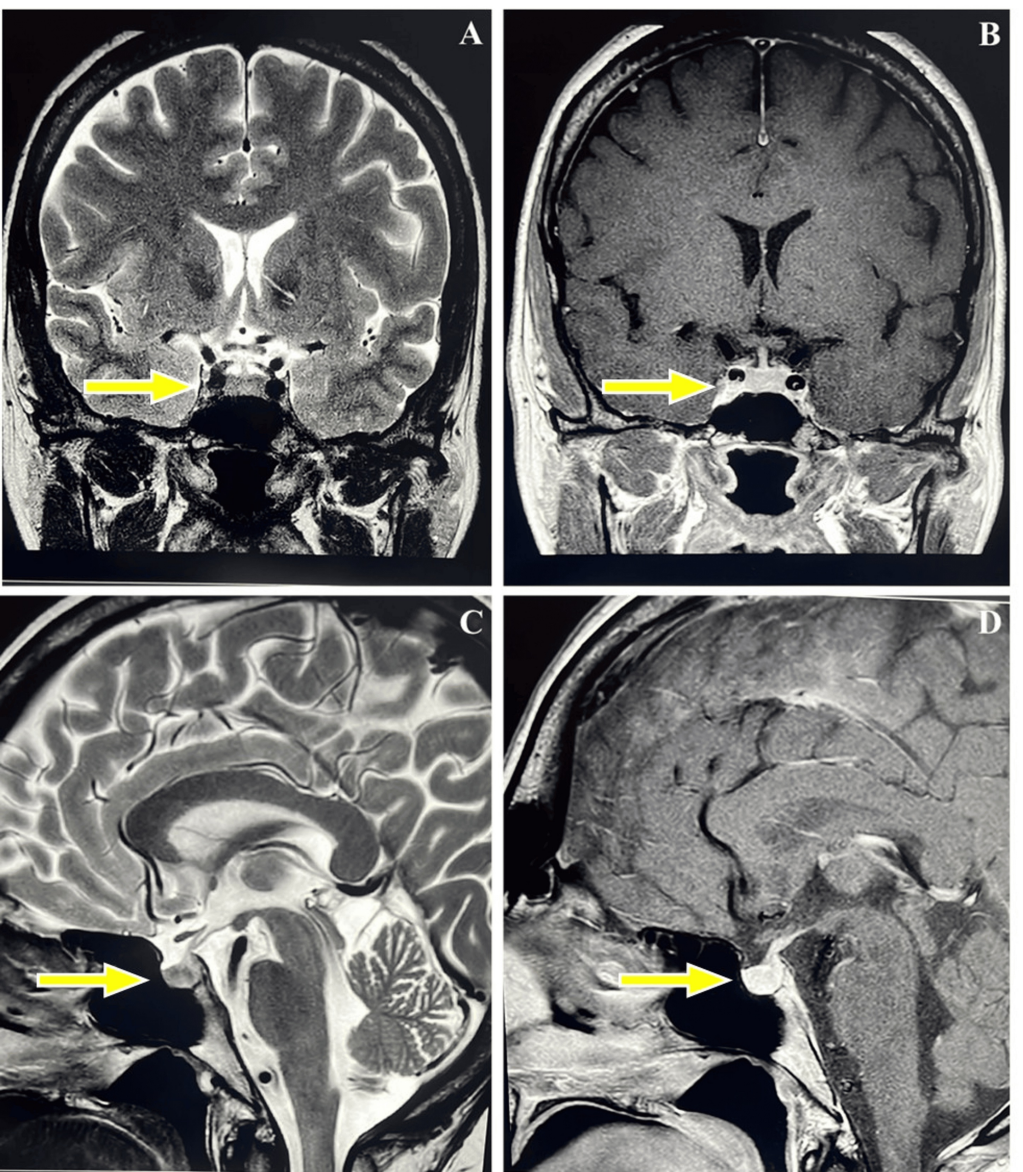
Normal MRI brain findings (TSE protocol, 2 mm slice thickness), confirming the absence of pituitary abnormalities such as mass lesions, stalk thickening, or gland enlargement TSE: turbo spin echo (A) T2 TSE coronal plane; (B) T1 TSE with contrast, coronal plane; (C) T2 TSE sagittal plane; (D) T2 TSE with contrast, sagittal plane The yellow arrow indicates the pituitary gland
